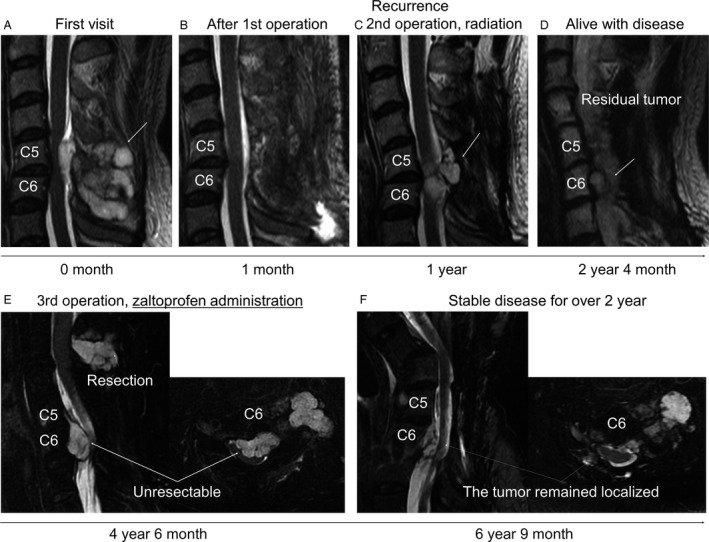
(A, B) Sagittal, MRI T2 weighted image of cervical spine at first visit on the initial hospital before (A) and after (B) the surgery. The tumor (arrow) was resected (1st operation), which markedly compressed the spinal cord. (C) Local recurrence was detected at C5‐6 and tumor excision (2nd operation) was then performed after 1 year from the initial diagnosis. An arrow indicates the tumor. (D) The residual tumor (arrow). (E) Sagittal, MRI T2 fat suppression image. The tumors markedly enlarged and severe pain occurred in his left hand. The tumor of C2‐4 was resected, while the tumor of C5‐6 was unresectable. Zaltoprofen (240 mg/day) was then administrated for the pain. (F) Before the final operation for severe cervical kyphosis, MRI T2 fat suppression image showed the localized tumor of C5‐C6 remained.
